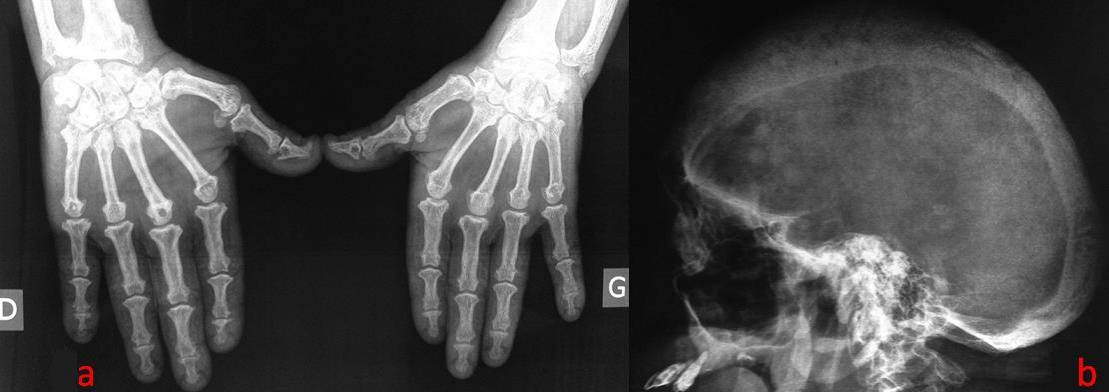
Association of radiological signs of acromegaly and hyperparathyroidism: (A) hands radiography: acromegaly: widening of the bases of distal phalanges, some hypertrophied terminal phalangeal tufts (spade appearance), some joint spaces minimally enlarged and premature osteoarthritis; hyperparathyroidism: triangular fibrocartilage complex chondrocalcinosis, acro-osteolysis of the 5th left phalangeal tufts and subperiosteal bone resorption; (B) lateral radiograph of skull: acromegaly: enlarged sella turcica with thickened skull vault and pneumosinus dilatans; hyperparathyroidism: pepper pot skull (salt and pepper sign)
